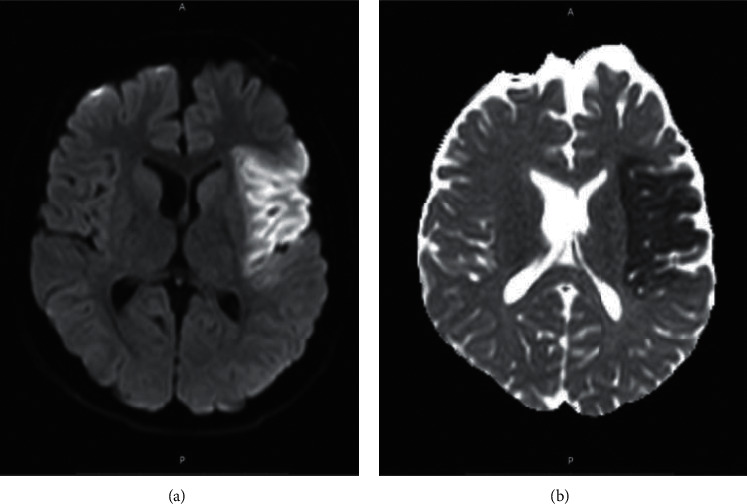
Axial MRI images (a) diffusion weighted and (b) apparent diffusion coefficient (ADC), showing acute brain ischemia in the territory of the medial cerebral artery.
